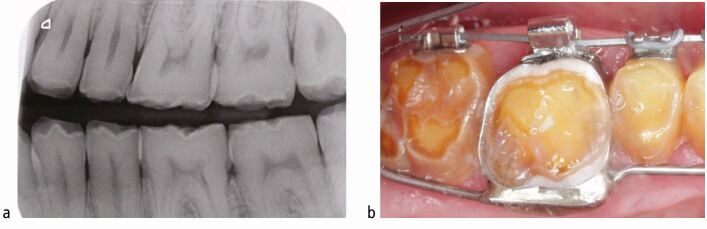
a, b) Example of a patient with amelogenesis imperfecta associated with reduced proximal enamel in the posterior teeth as seen on their bitewing radiograph. The band space will increase the available interproximal restorative space (on debond)
